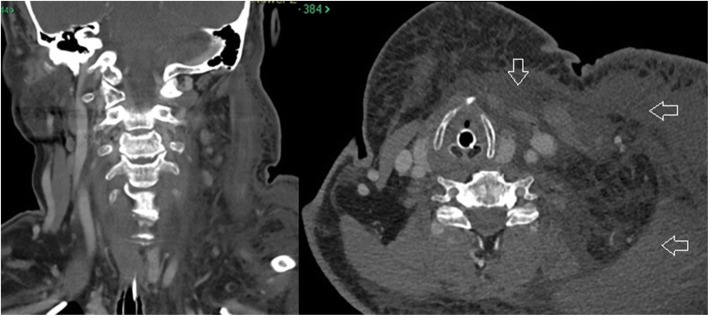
CT scan showing paratracheal edema
